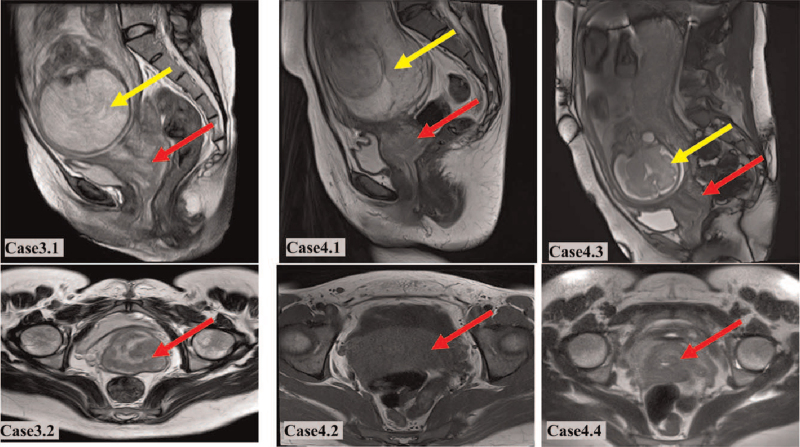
Case 3: T2 sagittal (3.1) and T2 coronal (3.2) pelvic magnetic resonance imaging (MRI) at 29 + 4 weeks of pregnancy reveals the fetus (yellow arrows) and tumor (red arrows). Case 4: T2 sagittal (4.1) and T2 coronal (4.2) pelvic MRI at 16 weeks and 29 weeks (4.3 and 4.4) of pregnancy reveals the fetus (yellow arrows) and tumor (red arrows).
